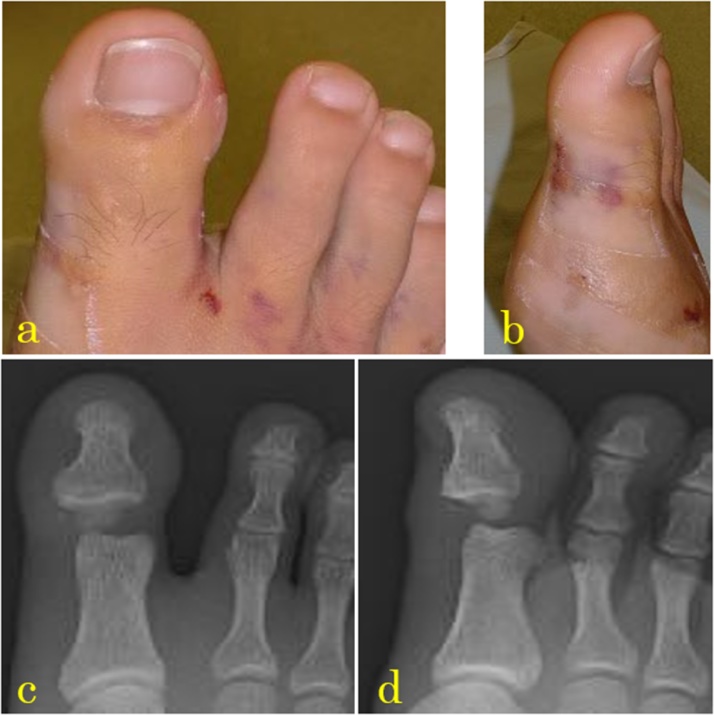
(a, b) After the failed closed reduction, the alignment was good, but the patient cannot move the joint voluntarily. (c, d) X-rays showing the sesamoid bone interposed in the IP joint.
